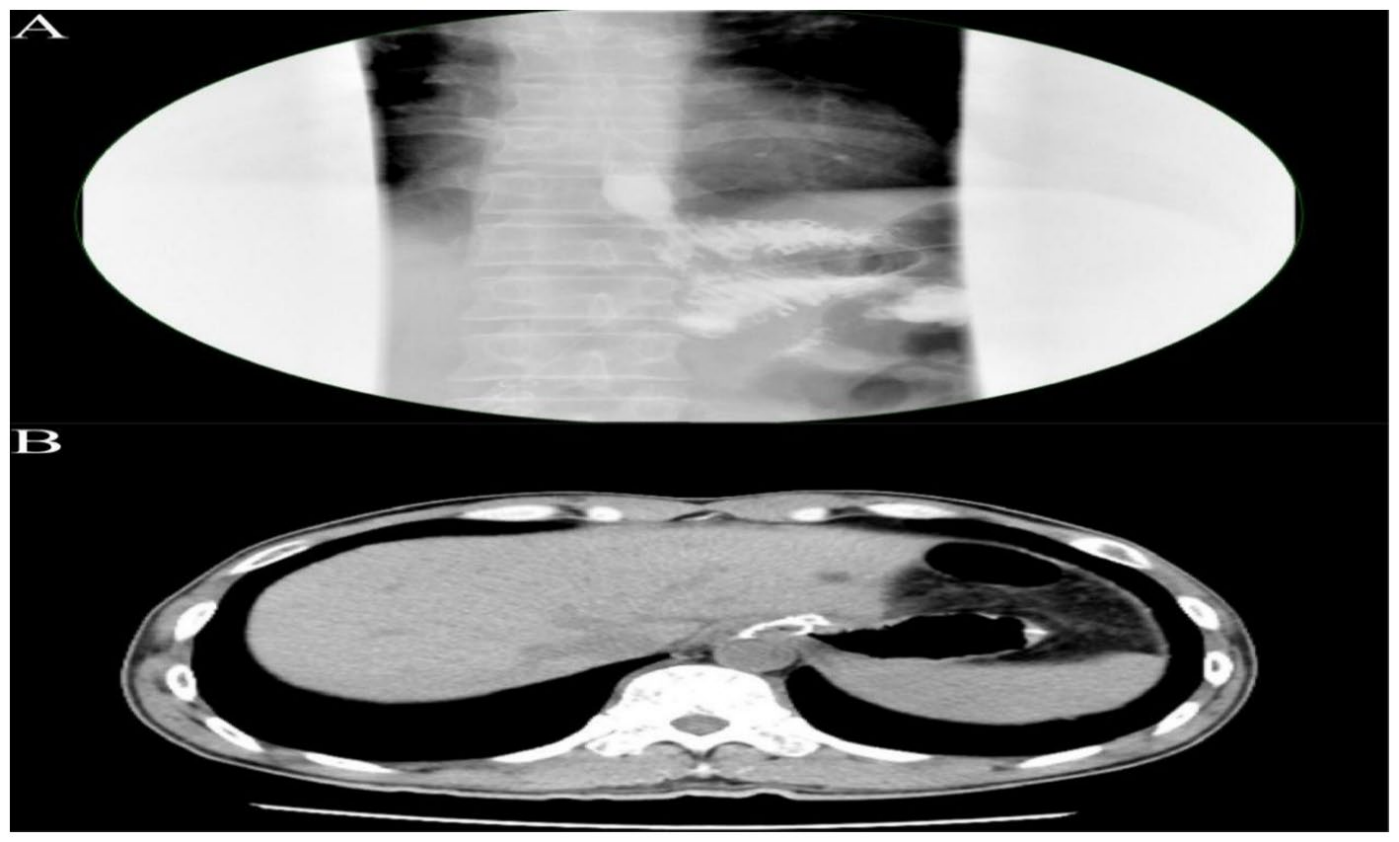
(A) Upper gastrointestinal contrast study demonstrating an esophagojejunostomy with an anastomotic diameter of approximately 0.6 cm. The contrast agent traverses slowly but smoothly, with no significant leakage observed around the anastomosis. (B) Abdominal CT scan reveals the esophagojejunostomy, showing a circumferential metallic density shadow, with the anastomosis appearing patent.
